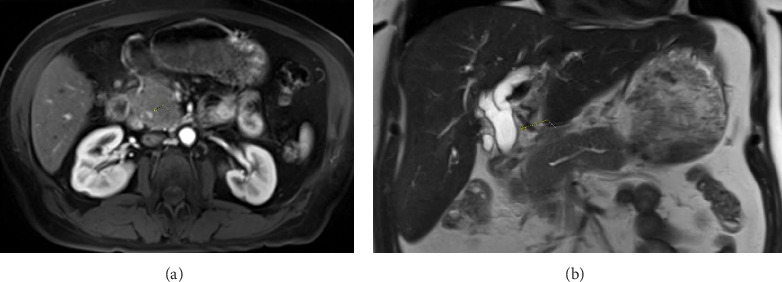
MRI MRCP without contrast. Irregular main pancreatic duct without dilatation (a). Extrahepatic biliary ductal dilatation of the pancreatic head/body fullness (b).
